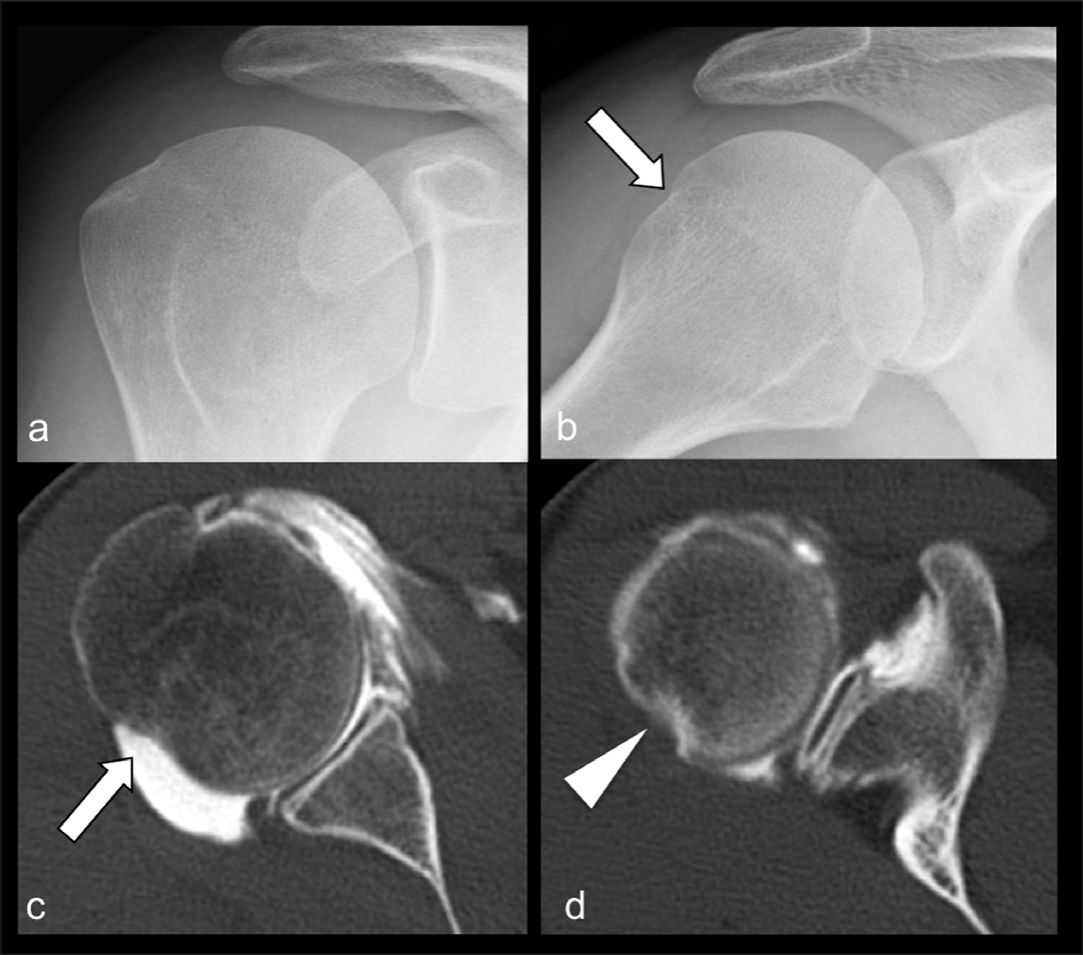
Frontal view of the shoulder in neutral rotation (a) and abduction/internal rotation (b) The depression (arrow) on the posterior head-neck junction of the humerus is a normal finding. The CT arthrogram axial image going through the mid third of the glenoid (c) shows the cortical depression with a large diameter base (arrow). The adjacent trabecular bone has a normal appearance. A CT arthrogram axial image through a more cranial part of the humeral head and the coracoid process (d) shows a depression with a smaller diameter base (arrowhead), corresponding to a Hill-Sachs lesion with sclerosis of the adjacent trabecular bone.
